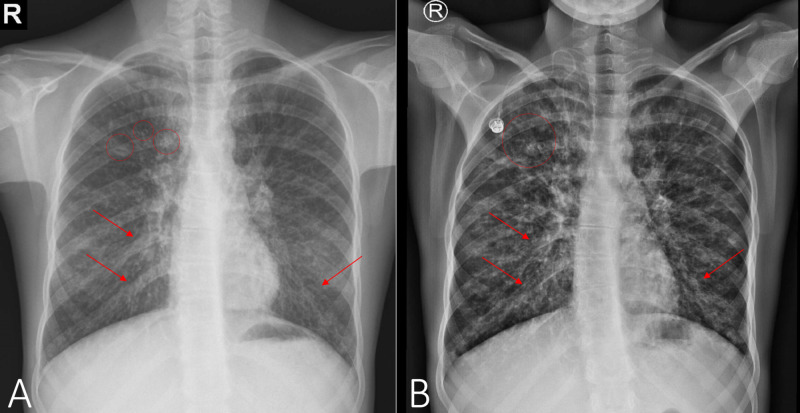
Chest radiography done in 2015 (A) and 2017 (B) showing worsening dilation of lower lobe bronchi (arrows) and area of signet-ring (circles) opacities in the right apical lung field
